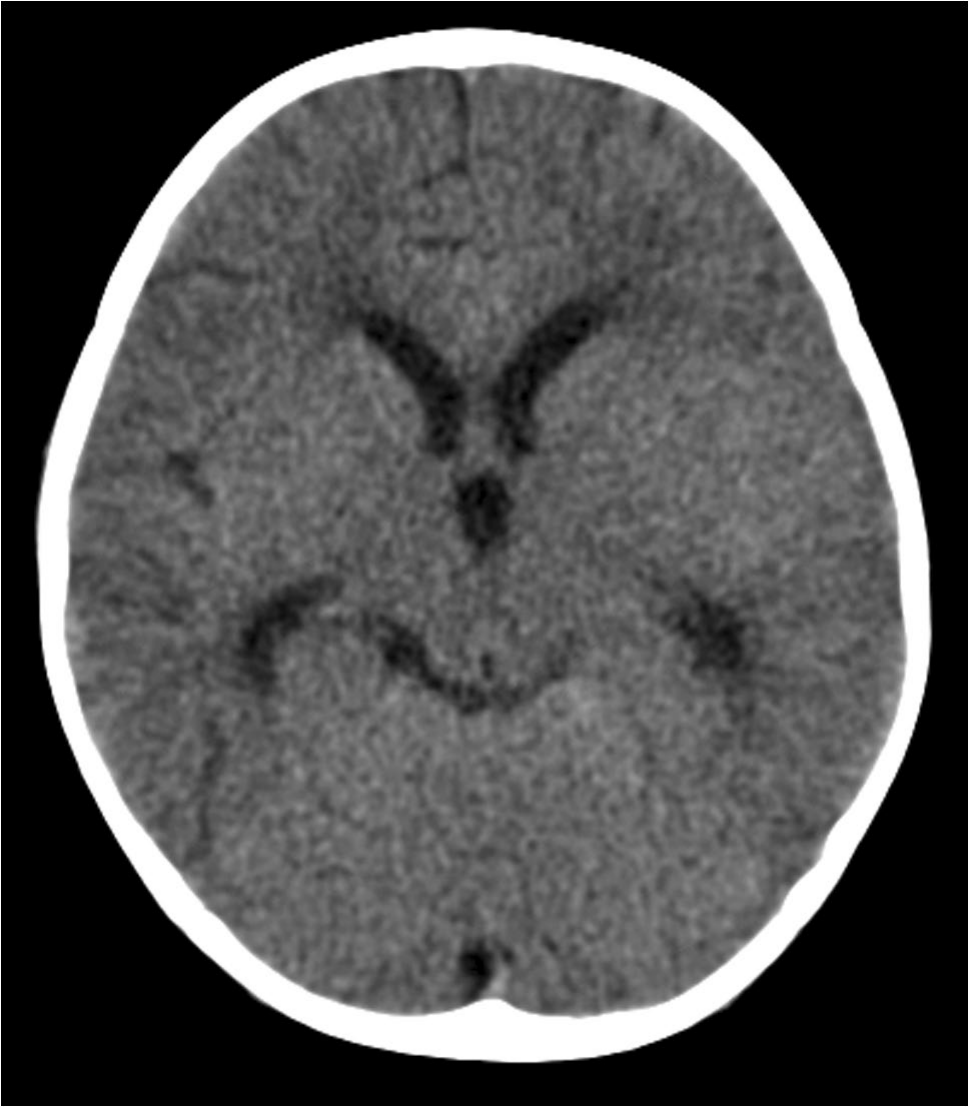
Axial non-contrast computed tomography of the  brain in a 4-year-and-6-month-old boy with tuberculous meningitis. There is only mild hydrocephalus despite the child being severely obtunded at presentation
